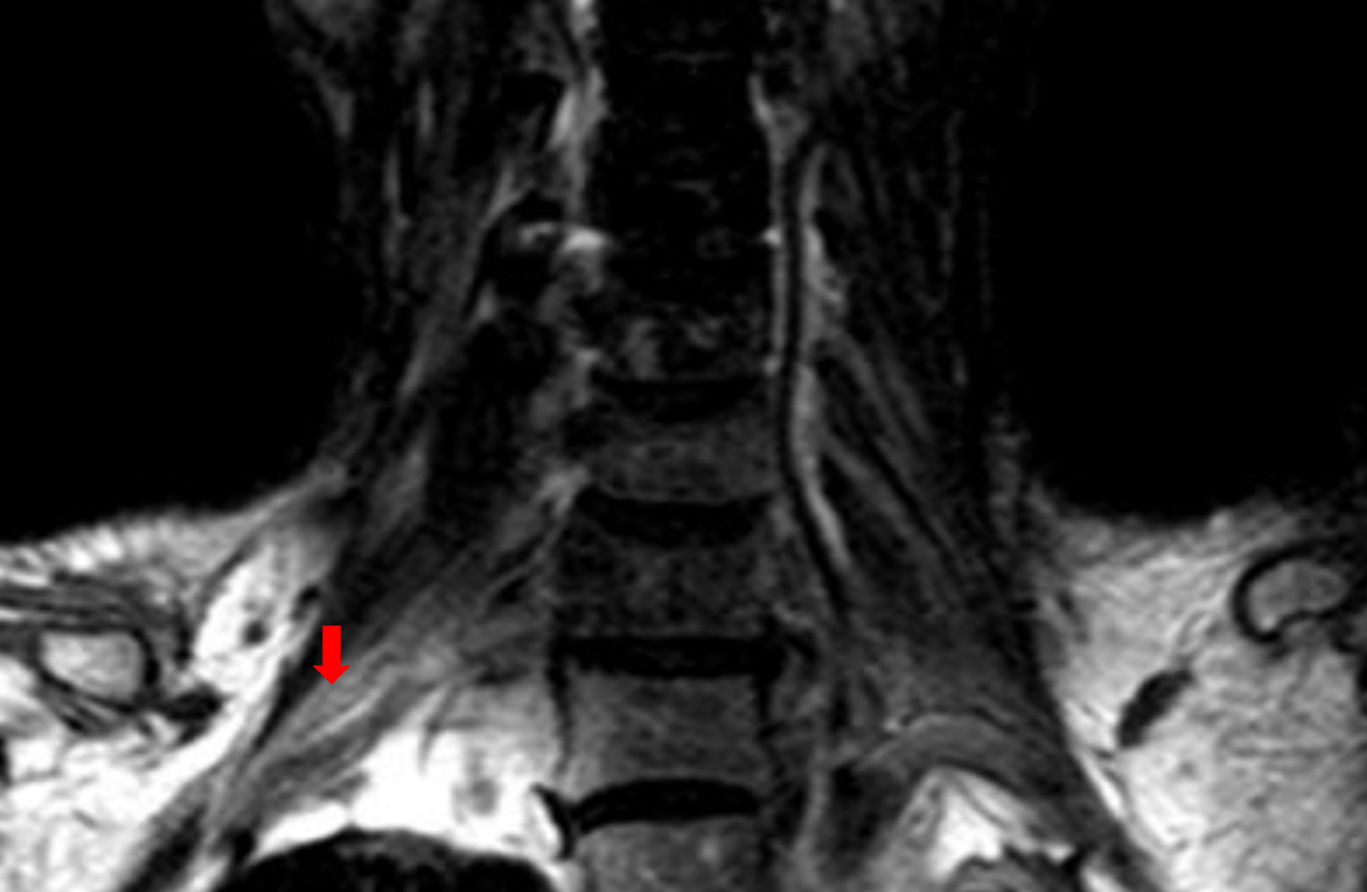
The T1-weighted coronal MRI scan of one case with unilateral RIBP The MR imaging showed high intensity within the left brachial plexus (red arrow), compared with the normal right brachial plexus.
